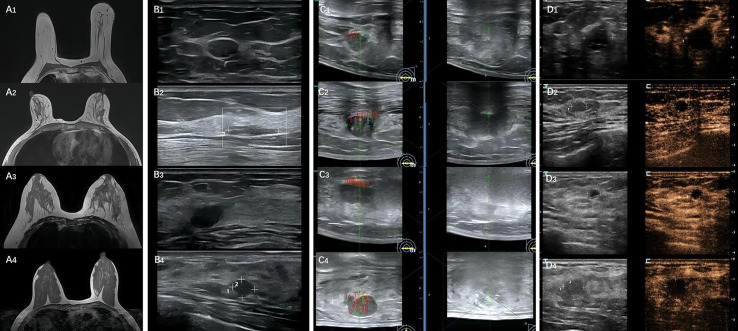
The entire process of HIFU ablation for different breast glandular types. (A1–A4) MRI images of four different breast types; (B1–B4) Ultrasound images of four different breast types; (C1–C4) HIFU intraoperative grayscale variation images for four different breast types (left side: preoperative, right side: immediate postoperative); (D1–D4) Immediate postoperative contrast-enhanced images of HIFU for four different breast types.
